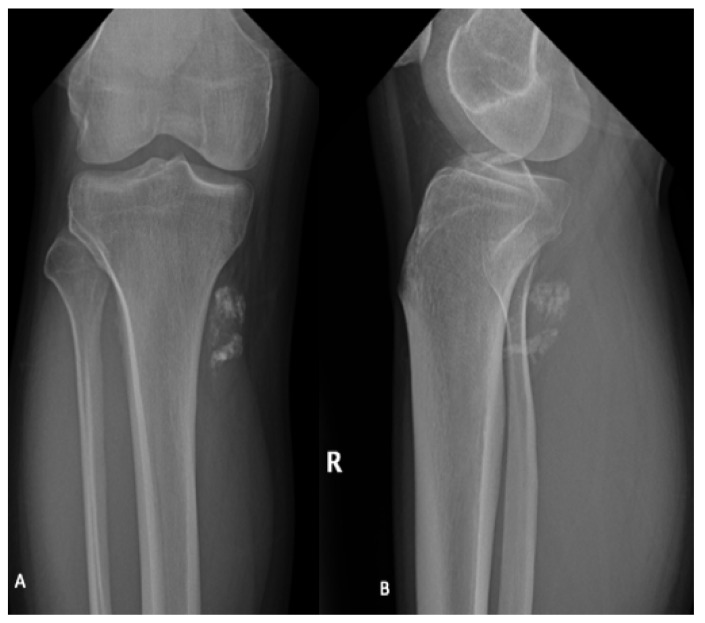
AP (A) and lateral (B) radiographs of the right (R) knee and lower leg in a 31-year-old male demonstrate coarse calcifications within the soft tissues adjacent to the posteromedial tibial plateau, corresponding to a biopsy proven synovial sarcoma. No significant articular abnormality. Adjacent bony structures appear unremarkable.
